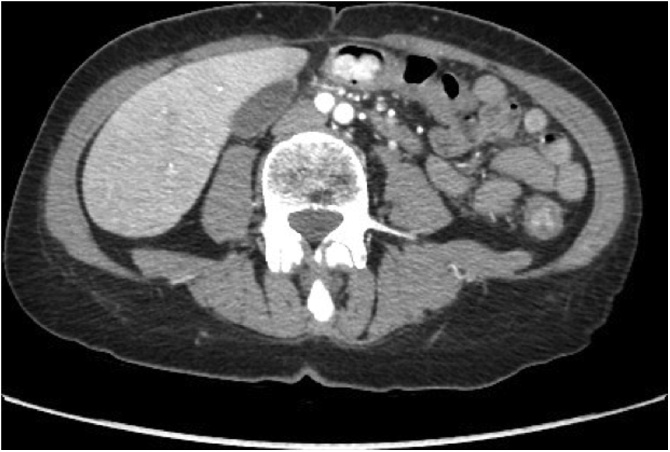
Computed tomography (CT) shows nodule formation of transverse colon with intense enhancement.
